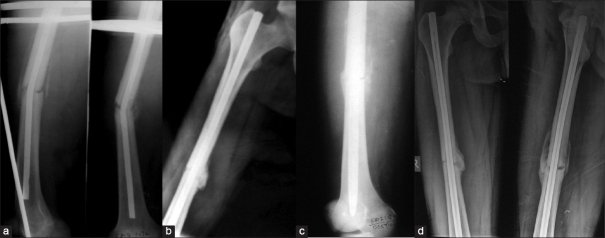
(a) Case of implant failure with bent K-nail; (b,c) postoperative radiographs after implant removal with well aligned fracture and expandable nail in place. (d) Follow up X-rays showing radiological union
